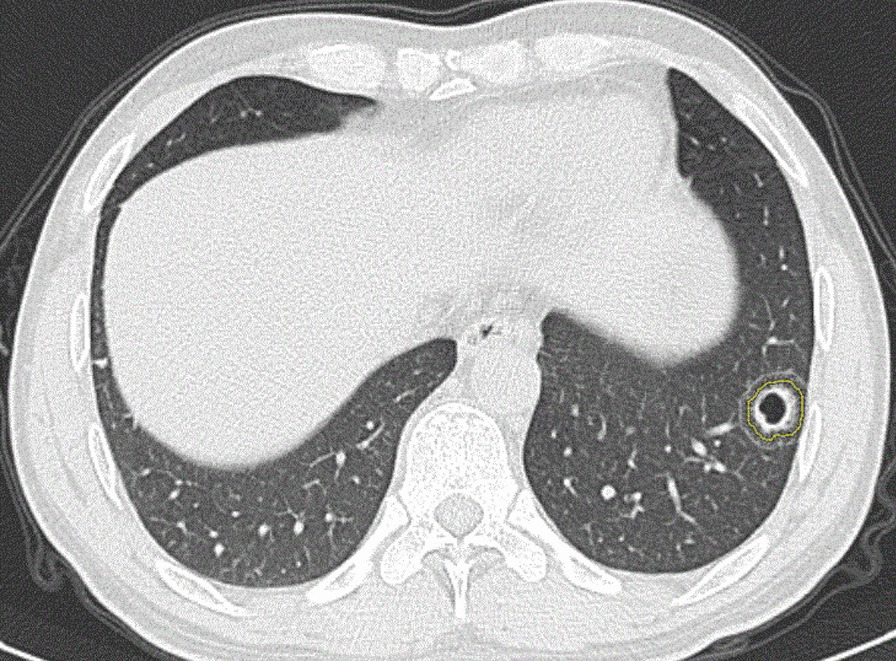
A 25-year-old male with AIDS complicated by Cryptococcus pneumonia presented to the hospital with fever for 2 weeks. A CT scan showed a thin-walled cavity in the lower lobe of the left lung. The automatic machine delineated the lesion approximately 1–2 mm from its outer edge
